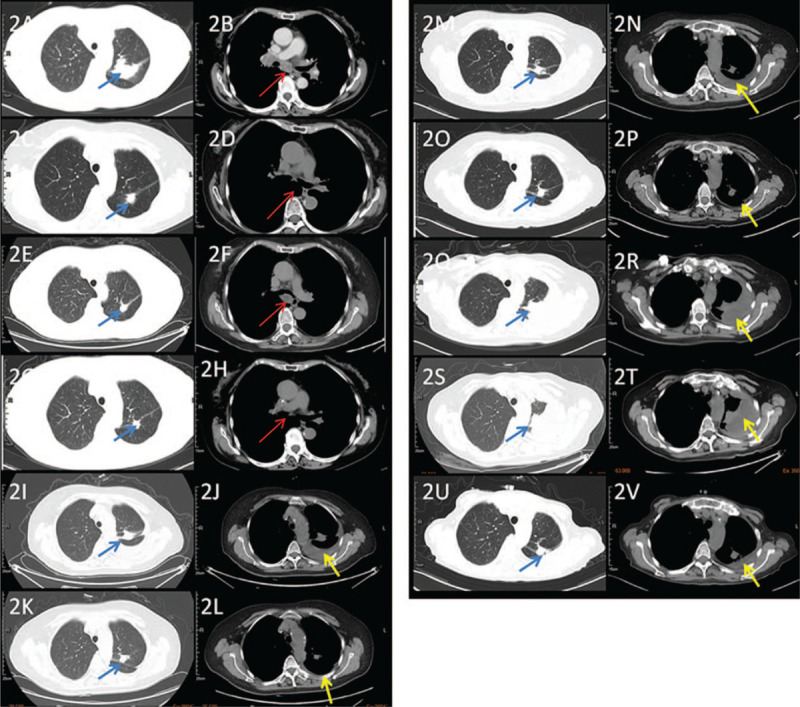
Computed tomography scans showing clinical response to treatments. The patient achieved partial remission after GEM/DDP (A–D). Progressive disease with enlarged subcarinal lymph nodes (F). The subcarinal lymph node disappeared 4 months after treatment with PEM/DDP (H). Lung lesions increased, and malignant pleural effusion developed in March 2016 (I and J). One month after gefitinib treatment, the patient achieved SD with shrinkage of the lung lesions and pleural effusion (K and L). After 25-month PFS, the volume of pleural effusion increased and a new lesion was found near the primary lesion (M and N), which disappeared after 2 cycles of PEM/CBP+BEV (O and P). The disease progressed with enlarged lesions and increased pleural effusion volume (Q and R). There was no benefit from the 2 cycles of DTX/DDP (S and T). Pleural effusion was reduced after osimertinib treatment (V). Blue arrows indicate the lesion, red arrows indicate the subcarinal lymph node, and yellow arrows indicate pleural effusion. GEM, gemcitabine; DDP, cisplatin; SD, stable disease; PEM, pemetrexed; CBP, carboplatin; BEV, bevacizumab; DTX, docetaxel.
